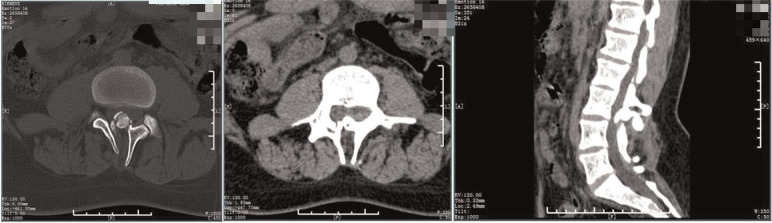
Preoperative CT. The lamina of the L3-5 vertebra were not fused, the spinous process of the L3 protrudes inward, considerating diastematorrhachia.
